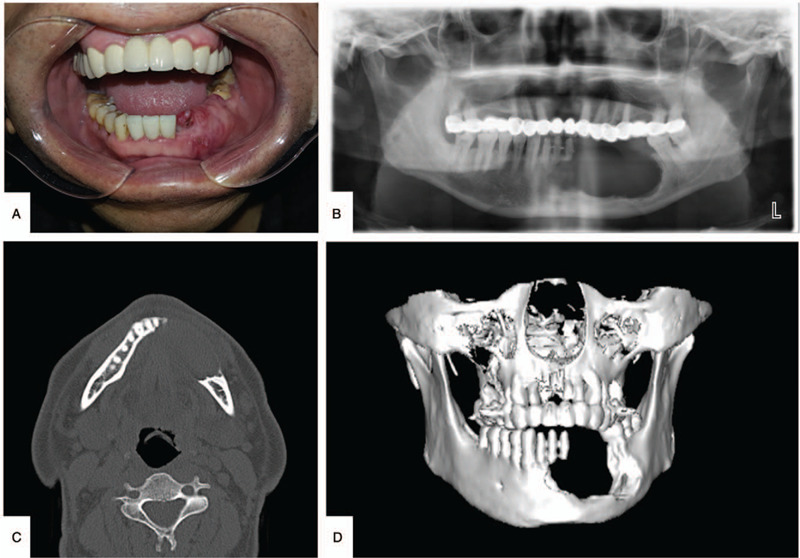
(A) Preoperative view of the intraoral mucosa of the patient with ameloblastoma; the surface of the oral mucosa was ruptured. Preoperative panoramic radiograph (B), horizontal CT scan (C), and 3-dimensional CT scan (D) showed an irregular radiolucent lesion of the mandibular body, exceeding the midline. CT = computed tomography.
